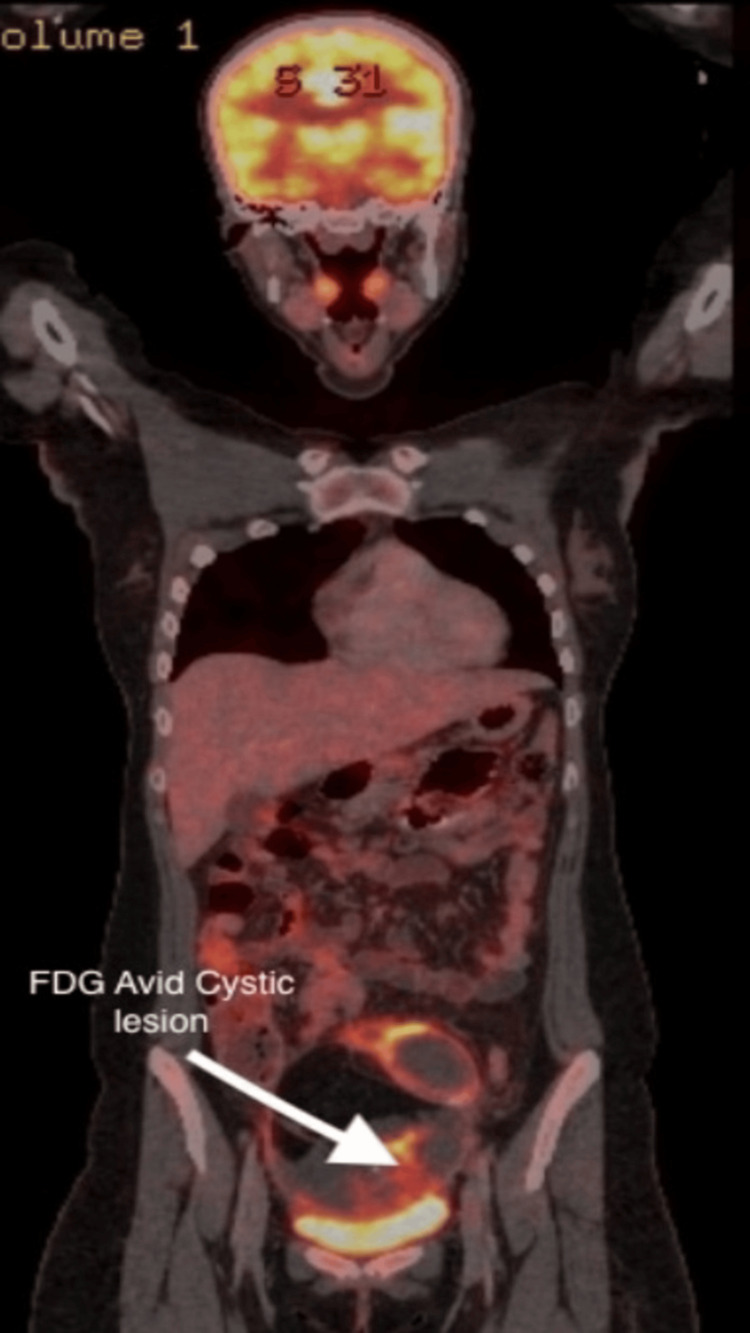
PET-CT scan of the whole body, coronal section, showing an FDG-avid lesion in the pelvis. The white arrow indicates a cystic lesion of the para-ovarian site. PET-CT: positron emission tomography–computed tomography, FDG: fluorodeoxyglucose.
